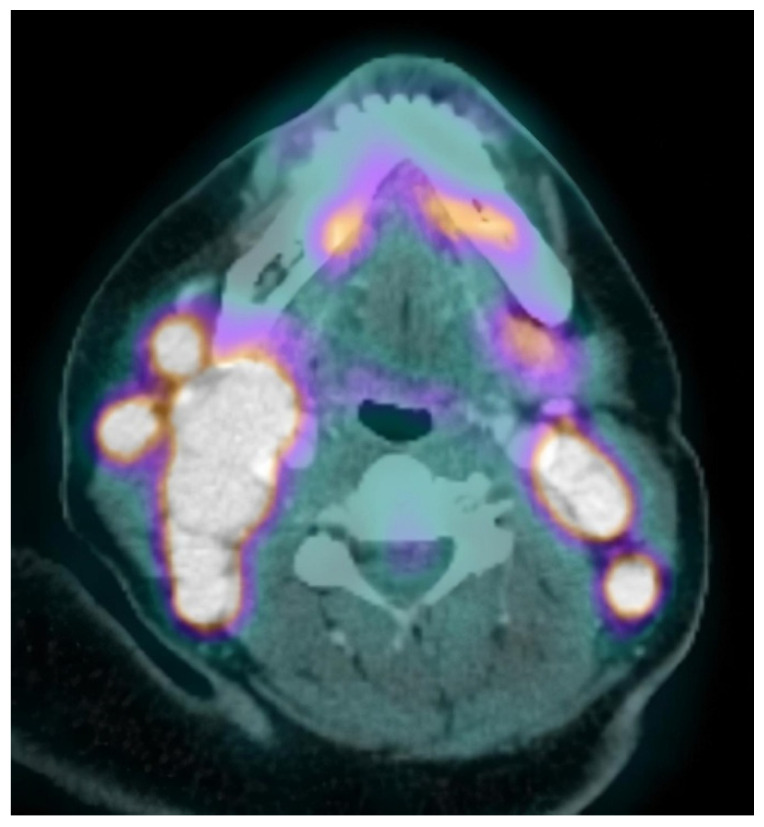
PET-CT: Pathological enhancement of several bilateral cervical lymphadenopathies.
